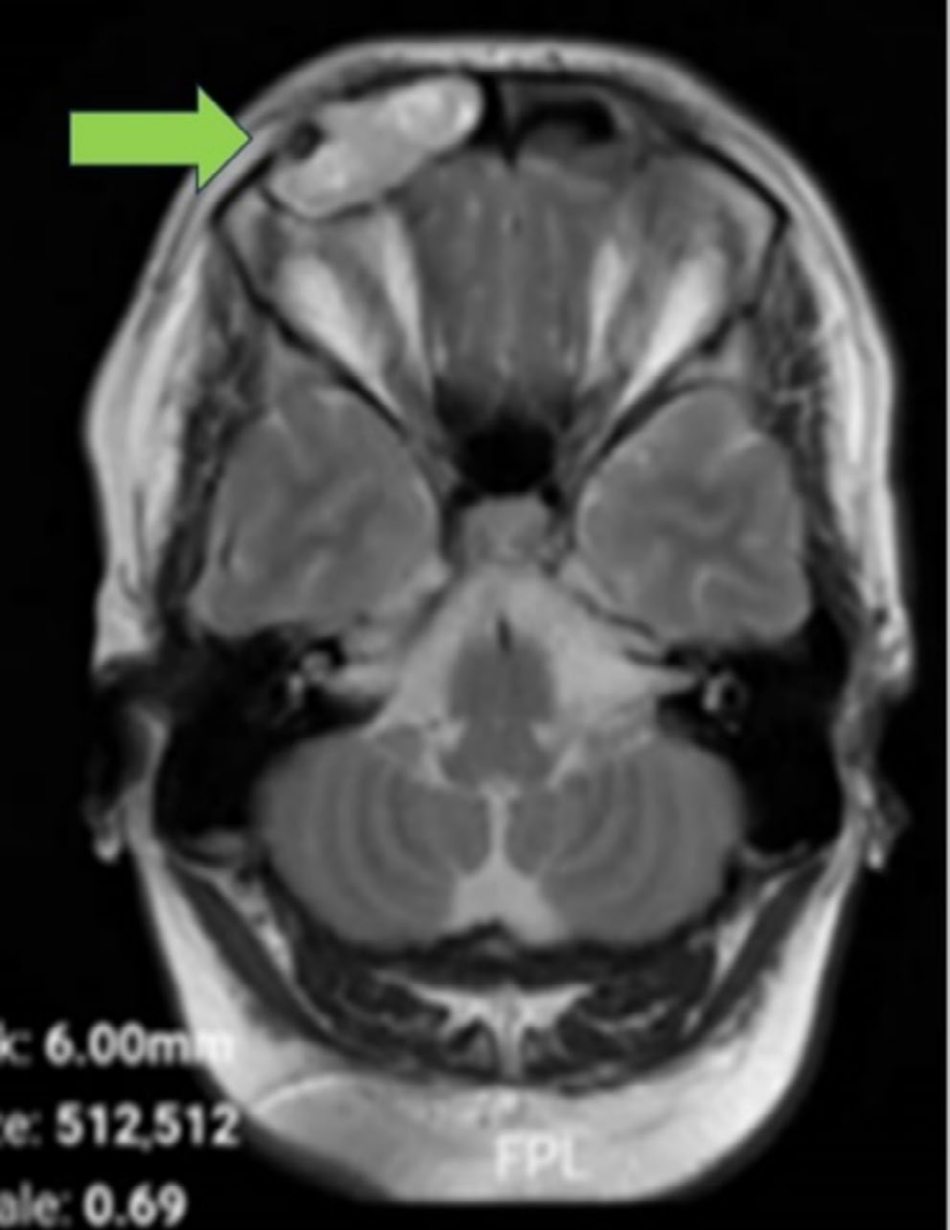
Axial T2‐weighted MRI showing a intermediate to mildly hyperintense lesion within the right frontal sinus, suggesting a cystic structure.
